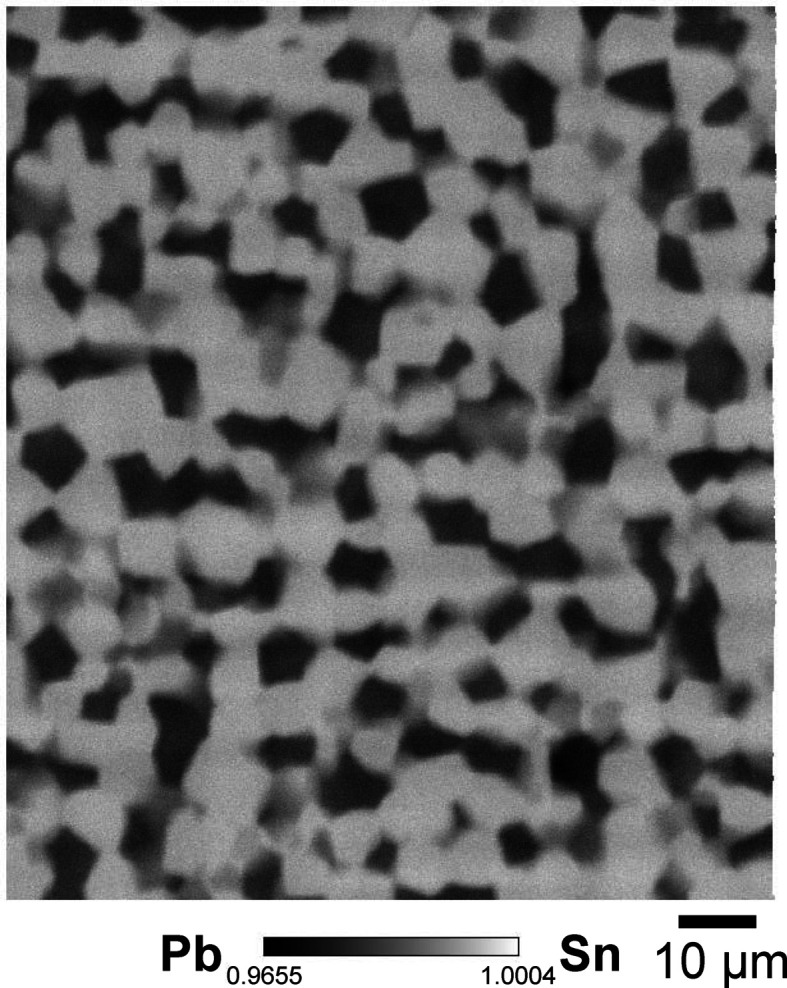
The scanning transmission image of Sn–Pb solder film. We used 100 keV X-rays with a focusing-beam size of 0.25 µm (V) × 0.26 µm (H). Eutectic grain structures, several micrometres in size, consisting of lead and tin, were clearly observed.
